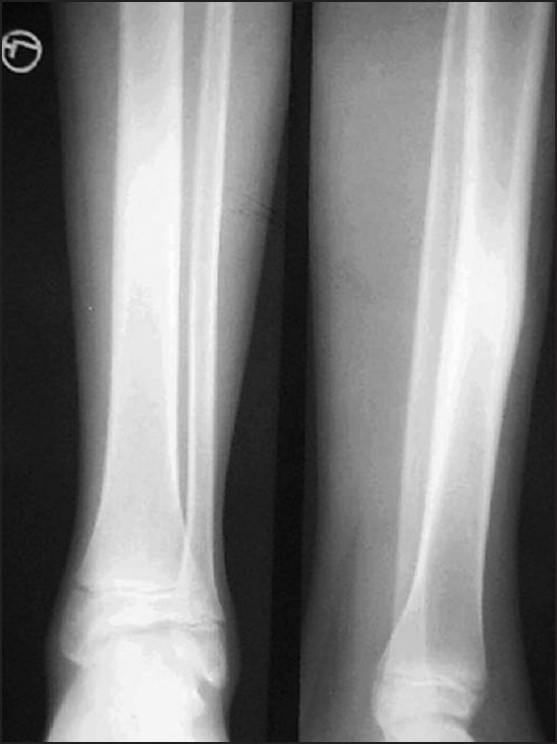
X-Ray of the right leg showing sclerosis on the medial aspect of the right tibia
